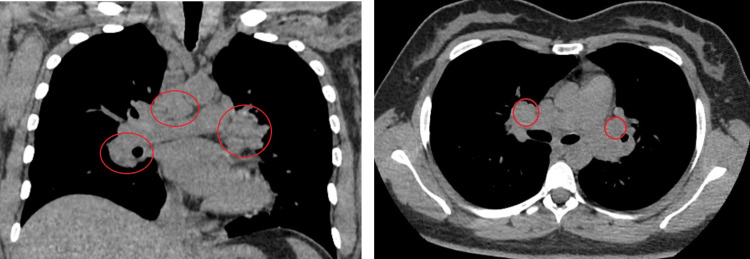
Chest CT scan showing bilateral hilar mediastinal adenopathy marked with a red circle
